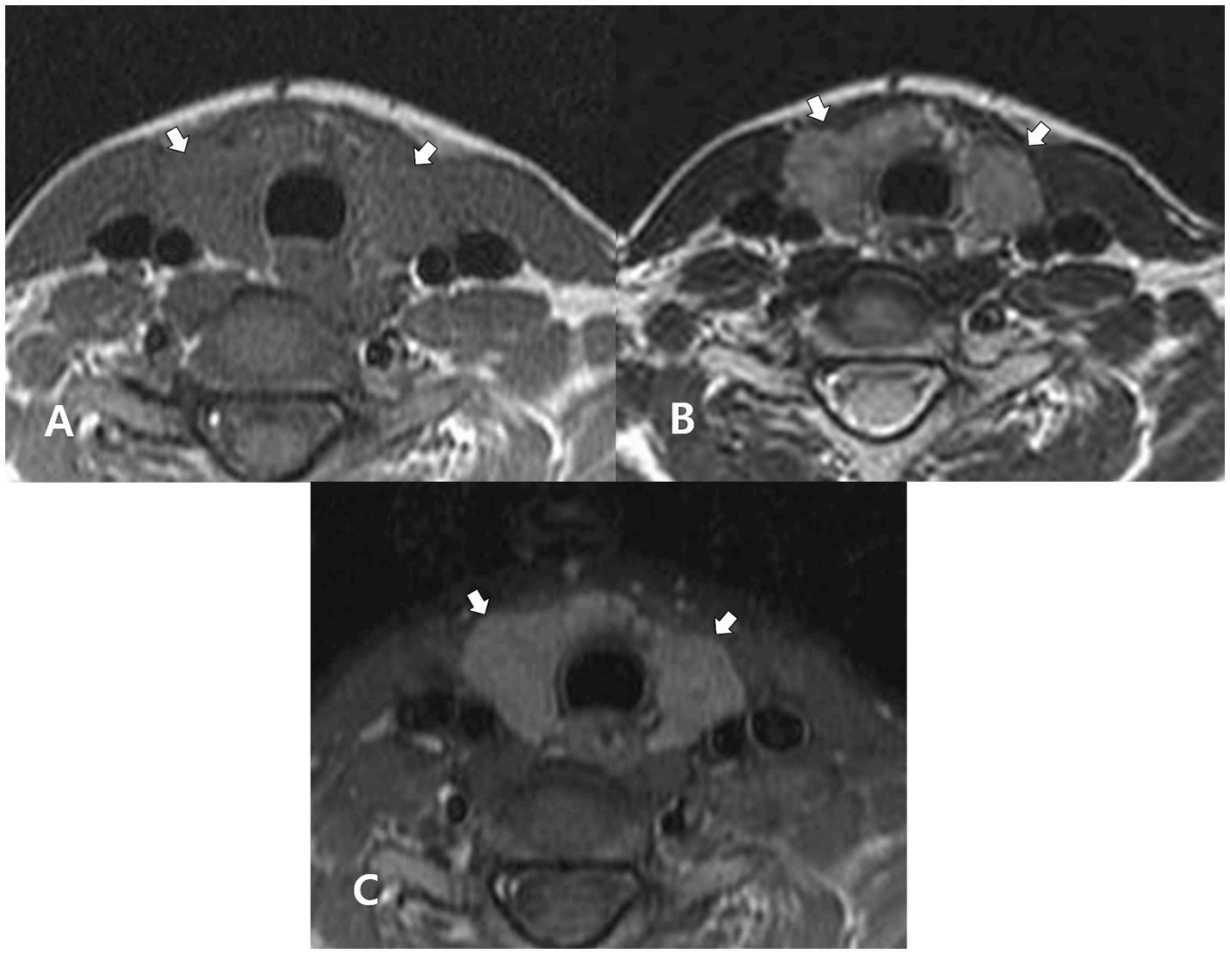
A 27-year-old woman with Hashimoto thyroiditis confirmed in cytology after ultrasound-guided fine-needle aspiration. In the non-enhanced axial T1- (A) and T2- (B) weighted images, the thyroid gland (arrows) exhibits homogeneous, iso-signal intensity, and inhomogeneous, high signal intensity, respectively, when compared with adjacent muscle. In both images, the thyroid gland (arrows) exhibits a normal gland size and smooth margin. In the enhanced axial, fat-suppression T1-weighted image (C), the thyroid gland (arrows) exhibits homogeneously increased enhancement, when compared with adjacent muscle.
